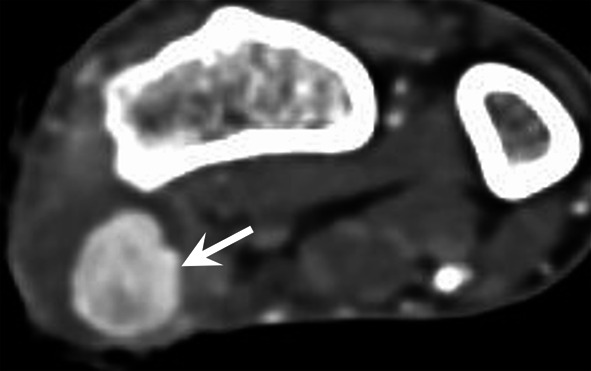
Pseudoaneurysm of the RA (arrow), after intra-arterial injection of buprenorphine, demonstrated with CTA
